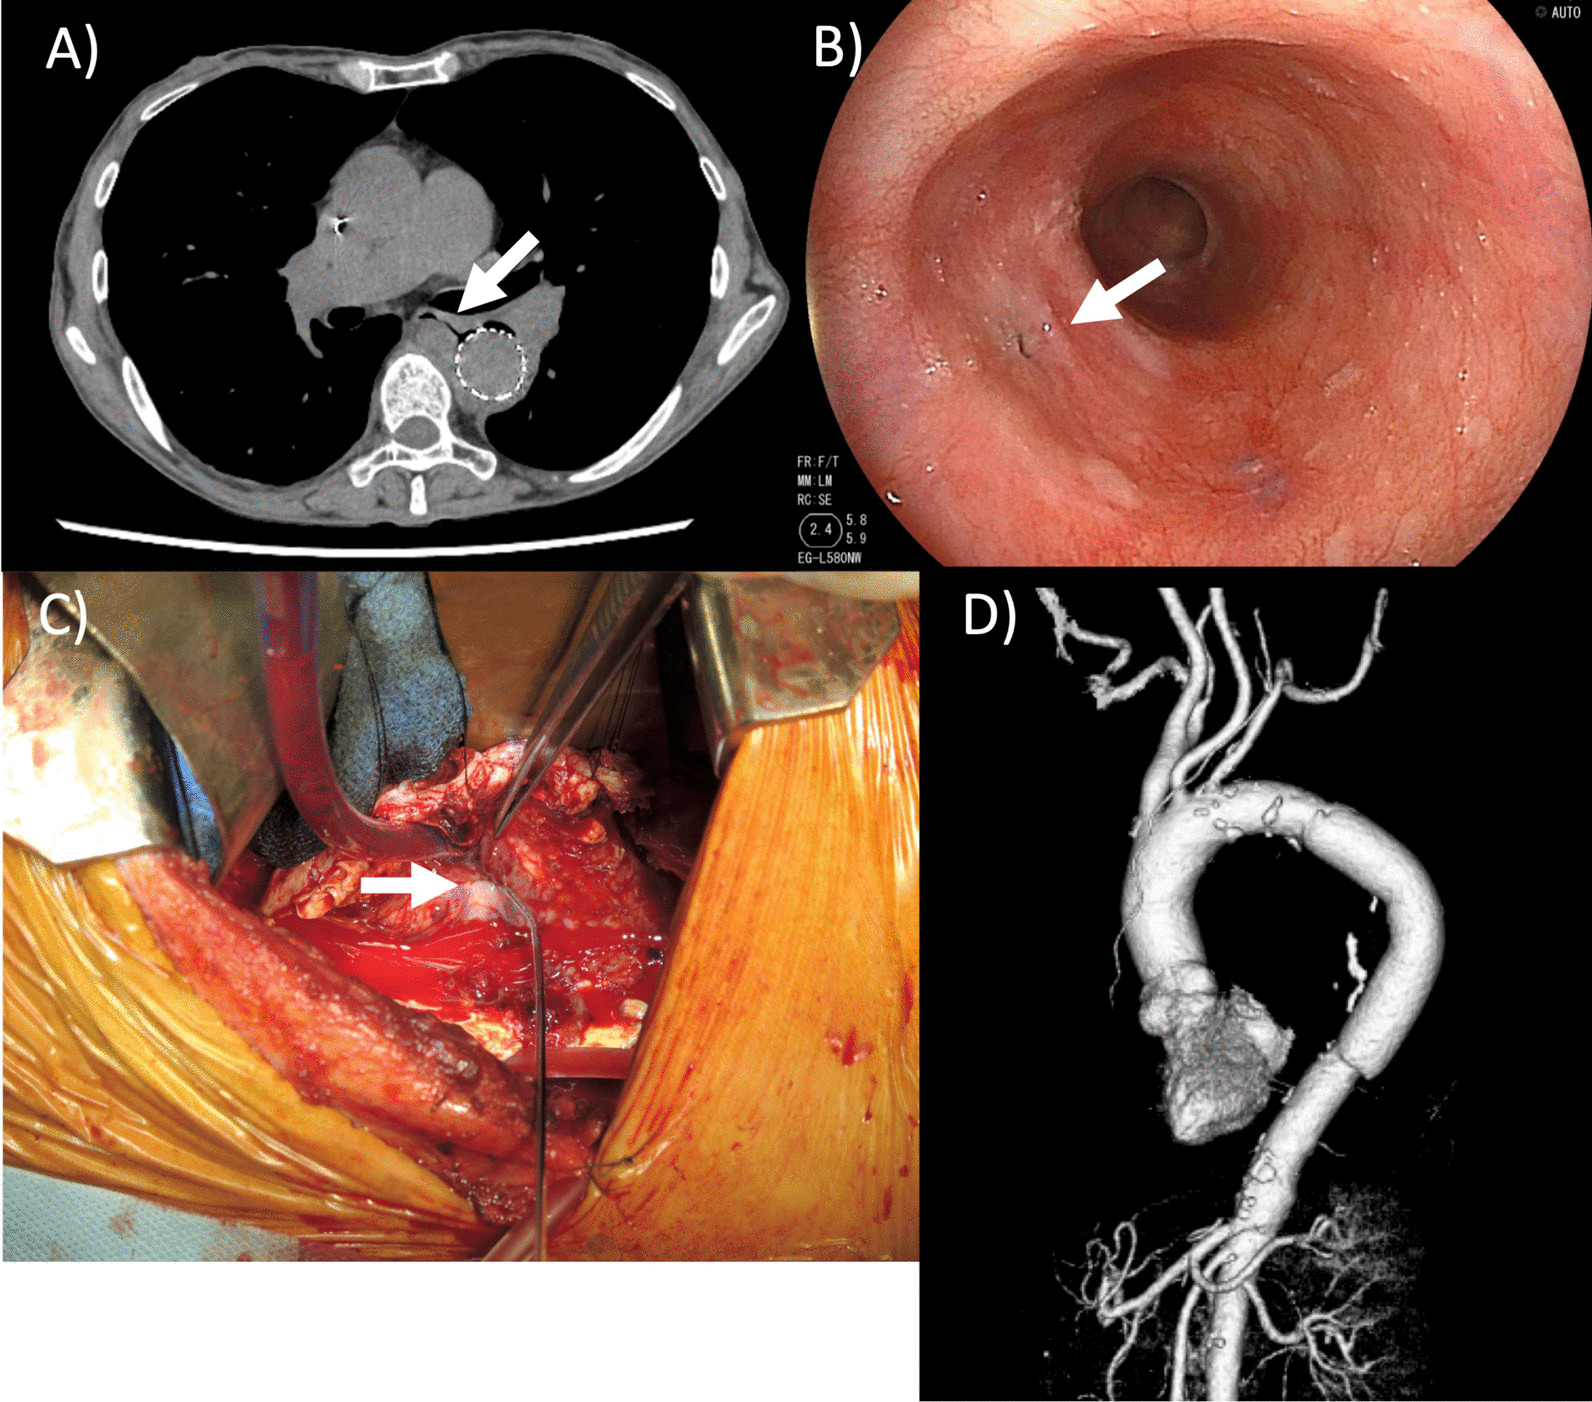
Postoperative computed tomography, endoscopy findings, intraoperative images, and postoperative computed tomography. a Computed tomography 2 weeks after thoracic endovascular aortic repair reveals air involving the esophagus to the periphery of the intra-aneurysmal stent-graft, suggesting the formation of an aortoesophageal fistula. b Upper gastrointestinal endoscopy 4 weeks after thoracic endovascular aortic repair shows that aneurysm-related compression of the esophageal lumen is relieved, but confirms an aortoesophageal fistula (arrow). c A fistula is confirmed in the lumen of the descending aorta. A 2-mm probe is passed through (arrow). d A three-dimensional computed tomography image after graft replacement. A blood vessel prosthesis is placed at a specific distance from the site of an aortoesophageal fistula
